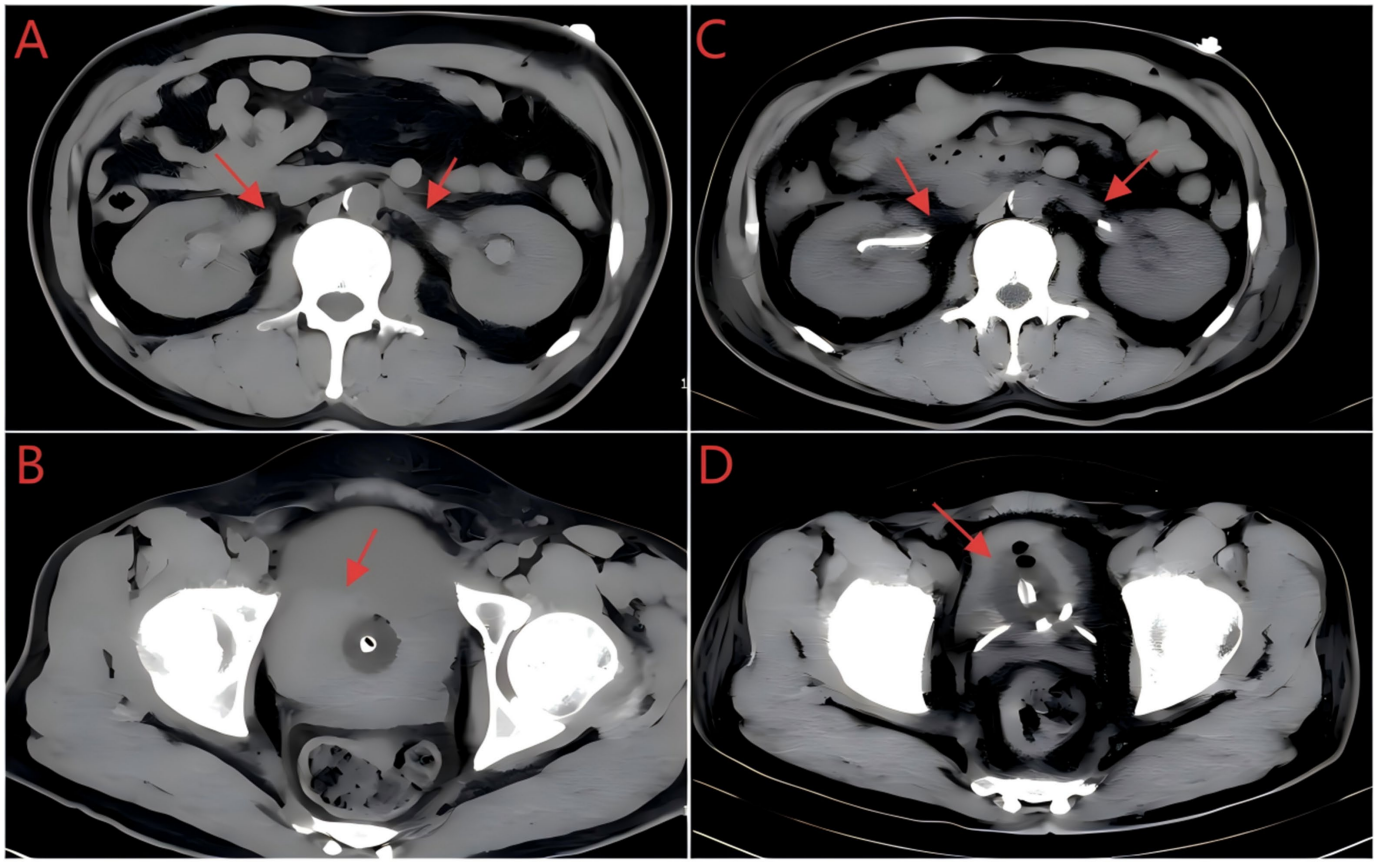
(A) Abdominal CT scan on admission (November 15, 2024) shows bilateral ureteral dilation with hydronephrosis, with red arrows indicating dilated ureters. (B) Abdominal CT scan on admission (November 15, 2024) reveals an overdistended bladder containing patchy hyperdense areas (red arrow) and an indwelling urinary catheter. (C) Repeat abdominal CT scan (November 17, 2024) demonstrates bilateral ureteral double-J stents *in situ* (red arrows), with mild dilation of bilateral renal pelvises and ureters. (D) Repeat abdominal CT scan (November 17, 2024) shows the indwelling urinary catheter within the bladder lumen, with residual small gas foci and minor patchy hyperdense areas.
